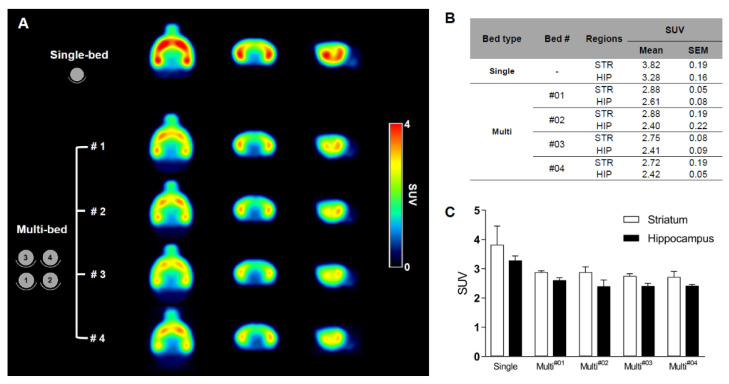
Summed ^18^F-FPEB brain PET images of the single-bed (n = 12) and each bed of the multi-bed system (A, n = 3 in each bed). Comparison of regional SUV values of single- and multi-bed systems (B,C). STR, striatum; HIP, hippocampus; SUV, standardized uptake value.
